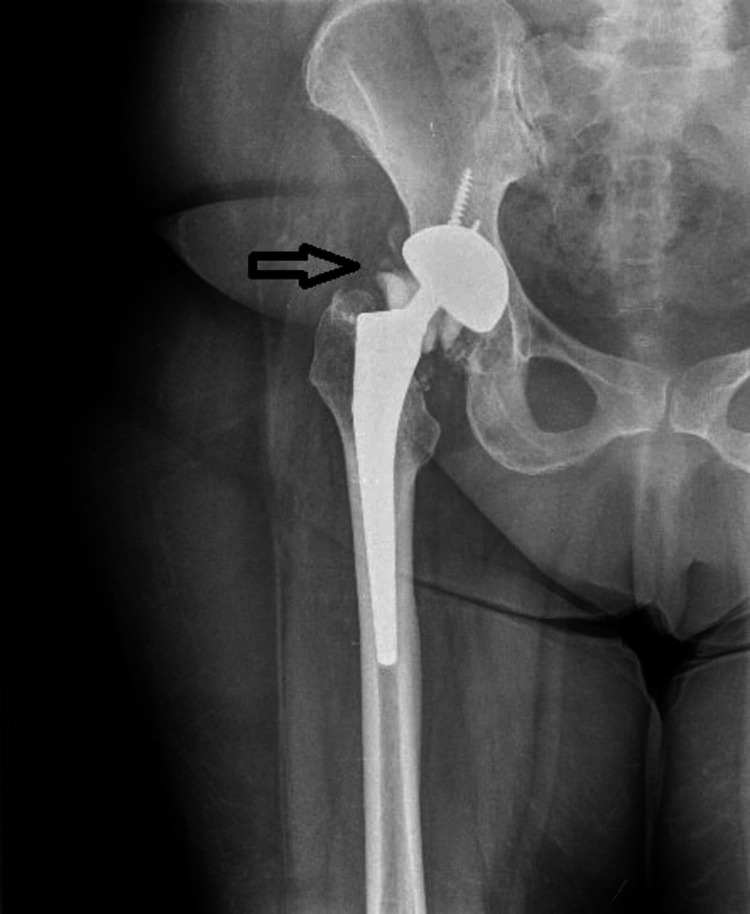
Anteroposterior hip radiograph demonstrates the fractured femoral head (black arrow). No osteolysis is presented.
